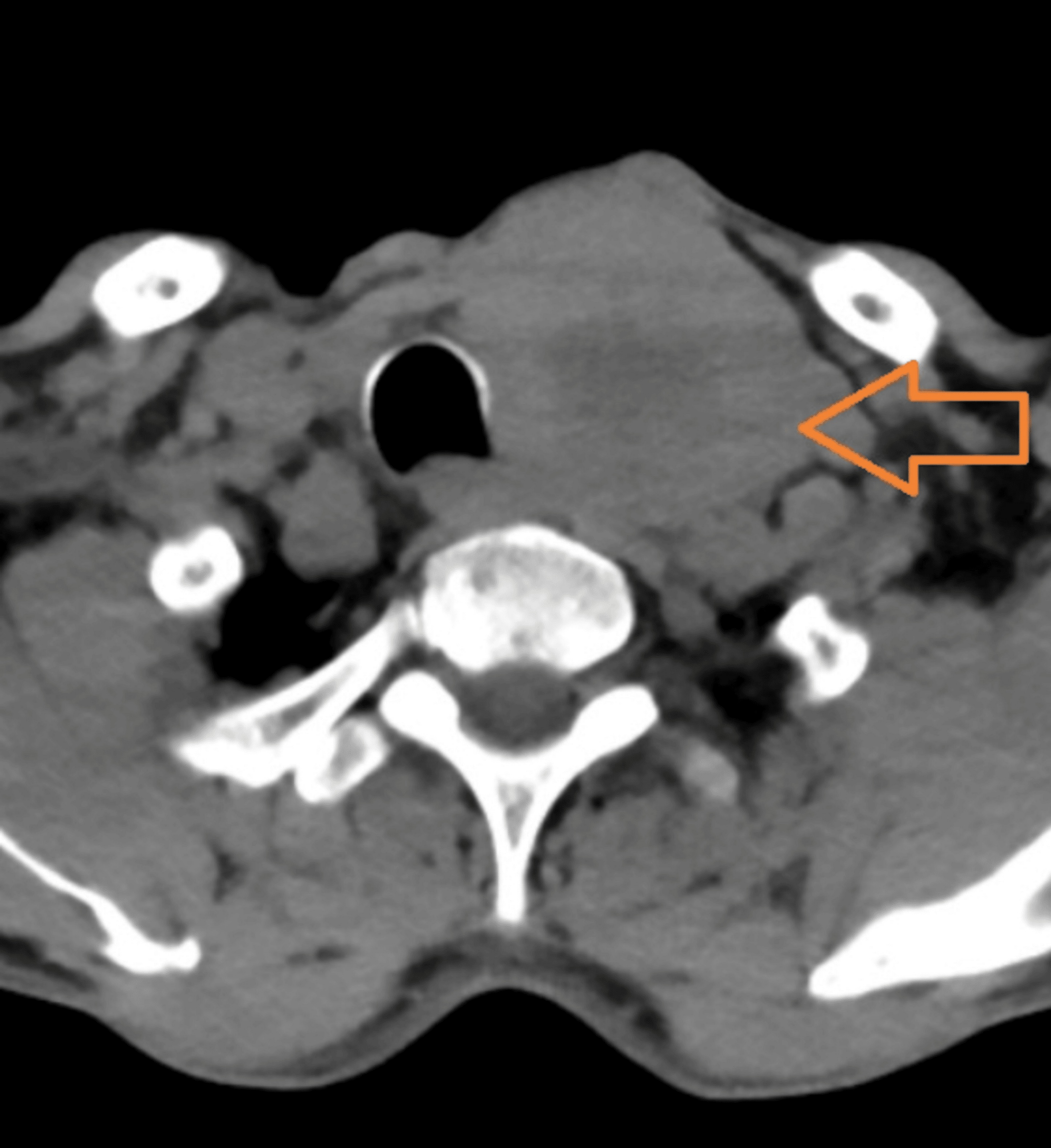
Contrast-enhanced computed tomography of the neck showing a malignant mass in the left thyroid gland measuring 31 x 27 x 26 mm (orange arrow).
